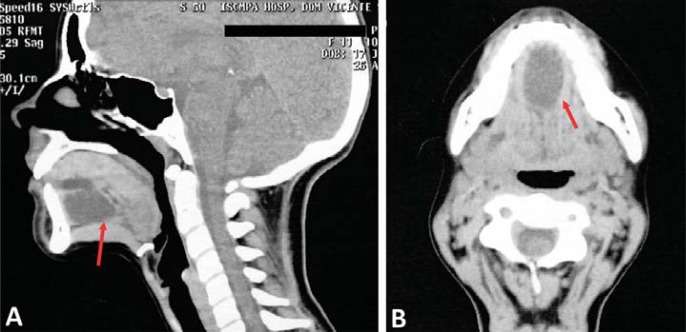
Pre-operative computed tomographic images. A – Sagittal scan with a well-defined unilocular cystic mass in the floor of the mouth (red arrows) that measured 3.8x2.5x2 cm (craniocaudal, anteroposterior, and transverse dimensions). B - Axial scan showing the lesion
